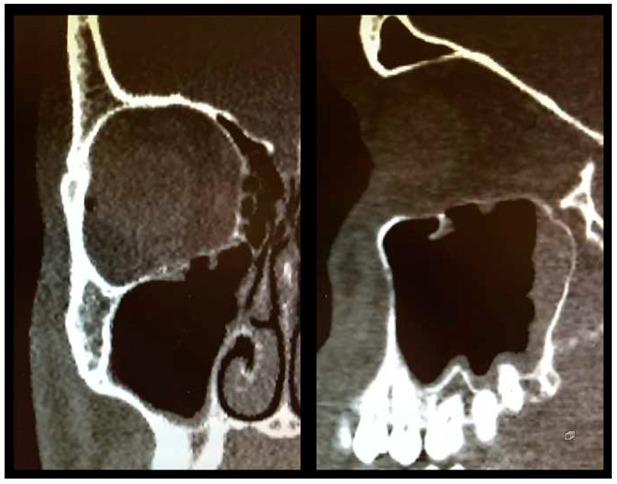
Postoperative CT scan following OFF reduction and placement of Ethisorb^®^ ((left) coronal view, (right) sagittal view).
